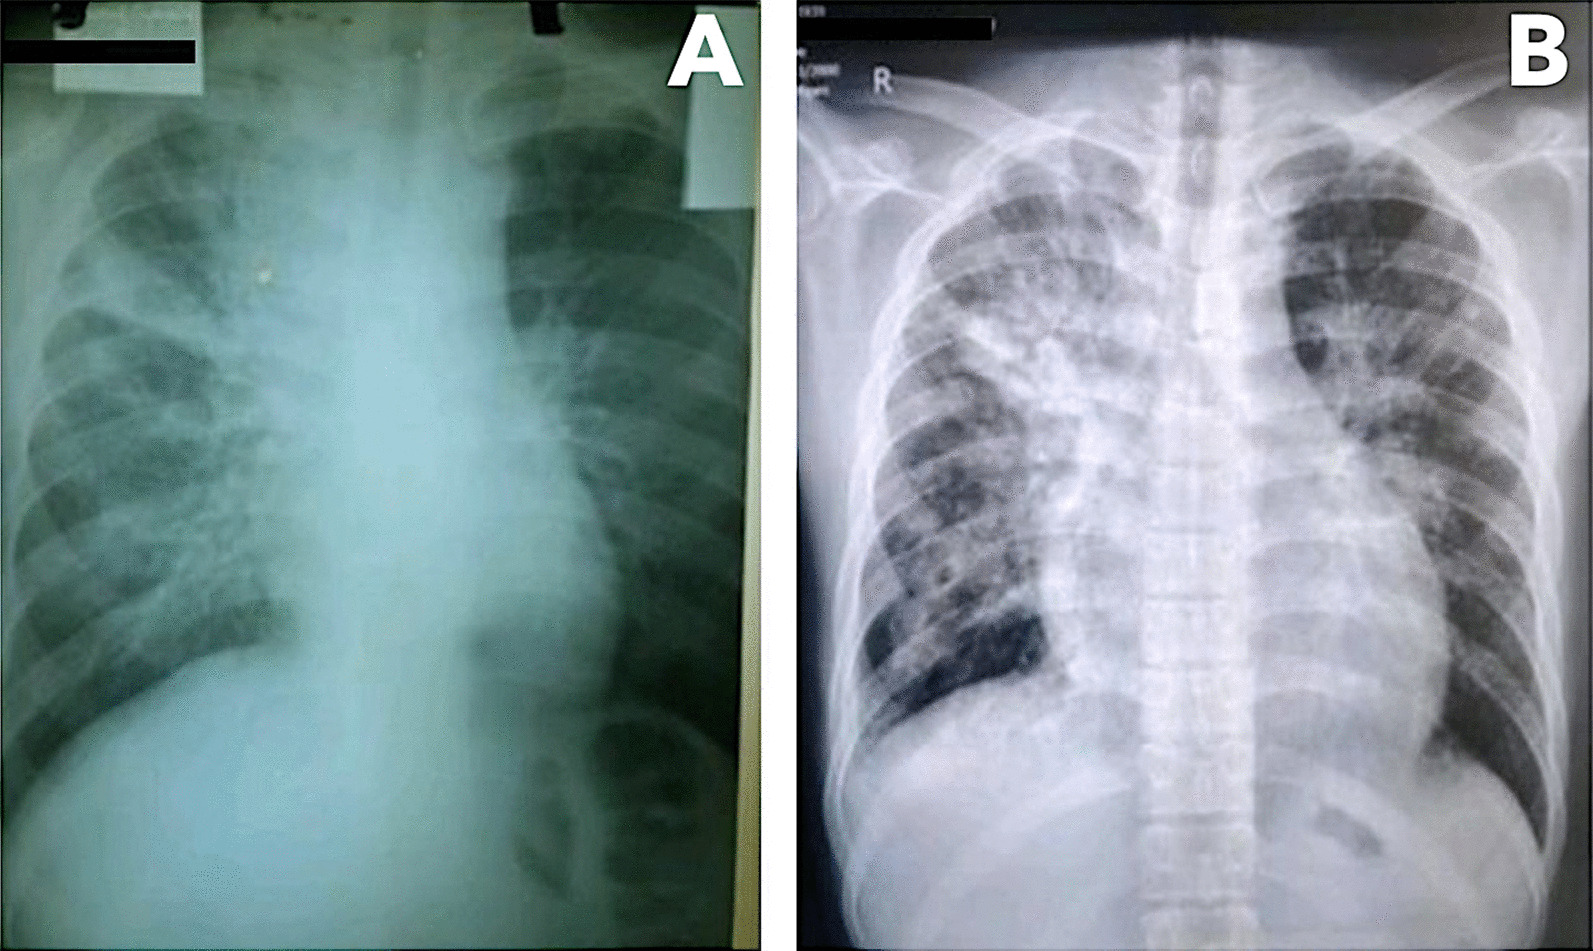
Chest radiographs taken 5 months prior to admission during initial diagnosis of tuberculosis (A) and taken on admission (B)
